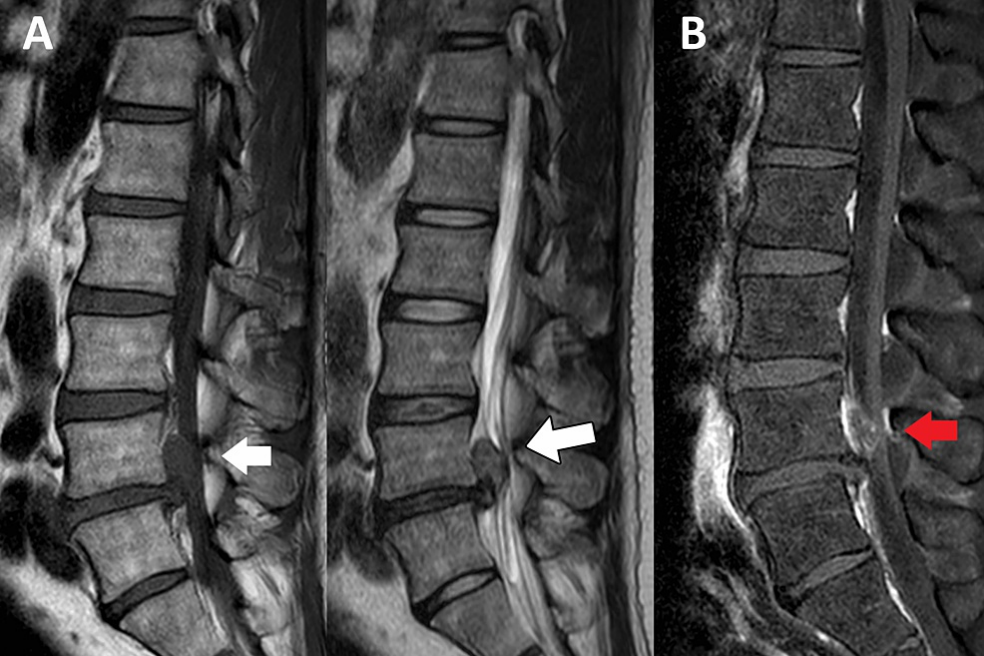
Magnetic resonance imaging of lumbar spine A) Magnetic resonance imaging of lumbar spine without contrast identifies a symmetric epidural mass lesion measuring 1.33 x 1.00 x 1.63cm at the left superior aspect of the L4/L5 disc. It shows T1W hypointense and T2W hyperintense signal (white arrow) and slight signal difference with the original disc. No bone erosion or destruction and the mass lesion compresses the spinal nerve cord. The differential diagnosis includes lumbar disc herniation and spinal tumor. B) Magnetic resonance imaging of lumbar spine with gadolinium contrast identifies that the L4/5 epidural mass lesion shows no contrast enhancement (red arrow) since disc is avascular. This implies the mass lesion is likely to be a superior migration of L4/5 disc herniation.
